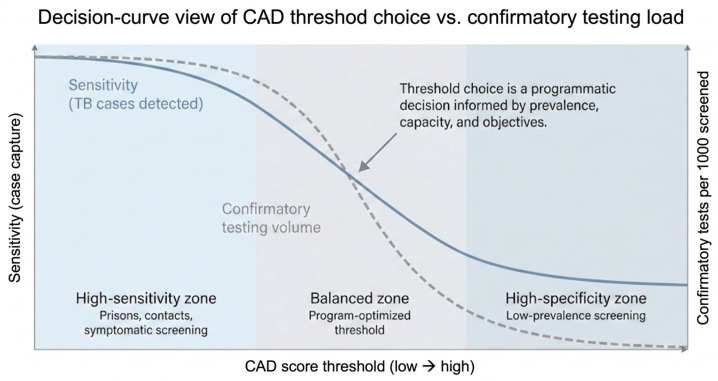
Decision-curve view of CAD threshold choice vs. confirmatory testing load. Computer-aided detection (CAD) systems for chest radiography allow programs to select an operating threshold that determines which individuals are referred for confirmatory tuberculosis testing. Lower thresholds prioritize sensitivity and maximize case capture but increase confirmatory testing volume, whereas higher thresholds reduce workload at the cost of missed cases. Optimal threshold selection is therefore a programmatic decision informed by local TB prevalence, screening objectives, and diagnostic capacity.
